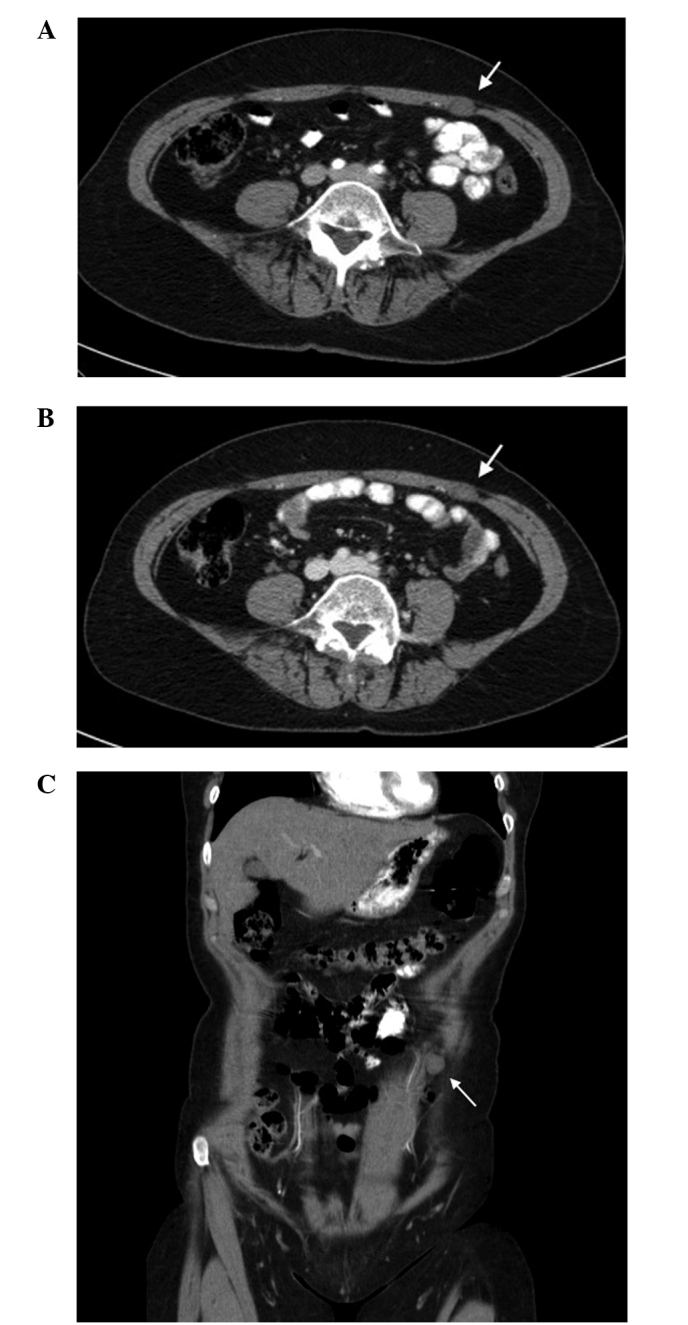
(A) Contrast enhanced-CT performed subsequent to IV administration of the contrast media. In the arterial phase, the mass exhibited a low enhancement and appeared slightly hypodense. (B) Contrast enhanced-CT performed subsequent to IV administration of the contrast media. In the venous phase, the mass remained slightly hypodense in comparison with the adjacent structures. (C) Coronal view of the contrast enhanced-CT. CT, computed tomography; IV, intravenous.
